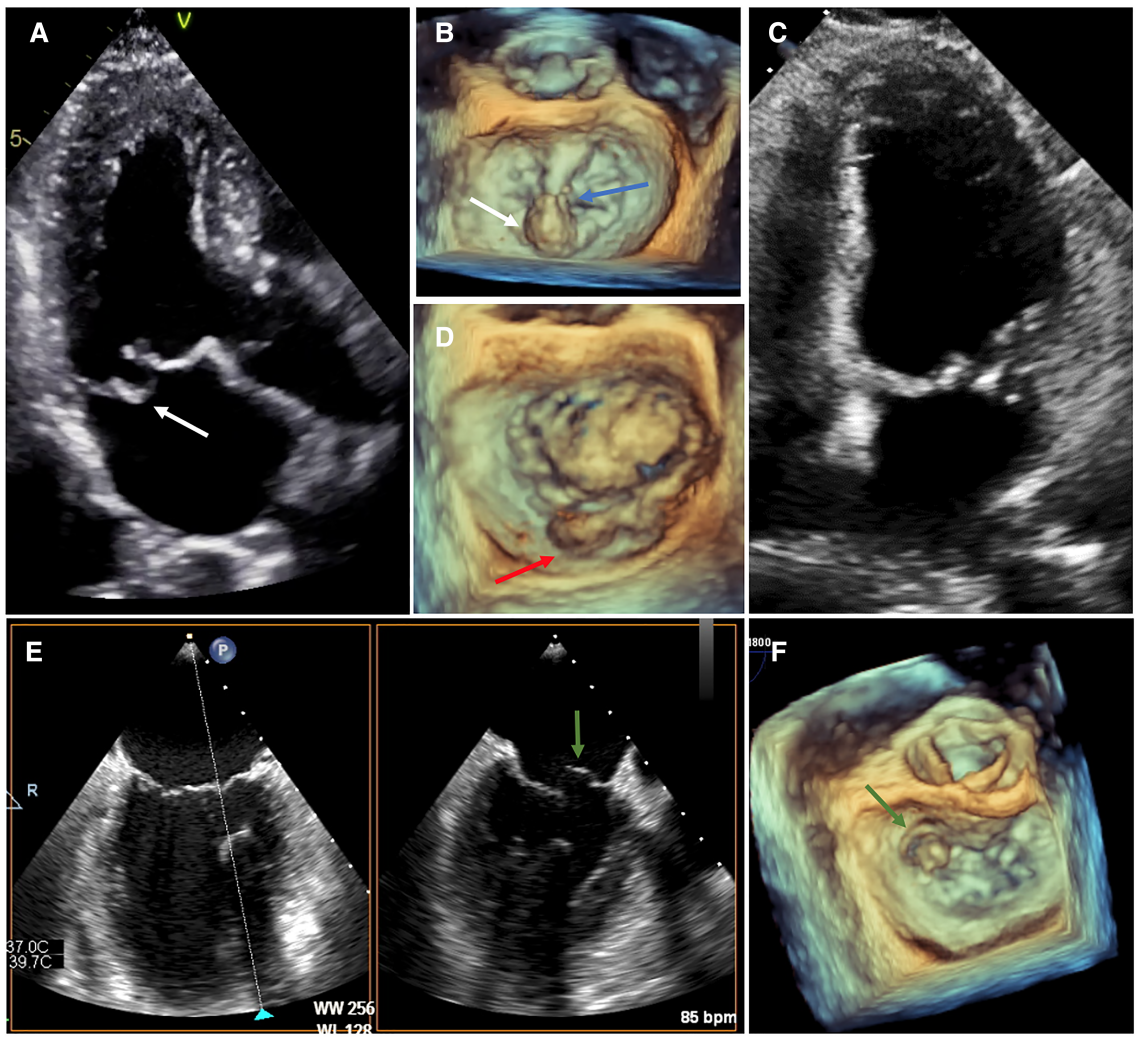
Morphological assessment of flail leaflet. (A,B) Patient with posterior flail leaflet visible on TTE (the tip is directed towards the LA). TEE confirms the involvement of segment P2 (white arrow) with chordae rupture (blue arrow). (C,D) Patient with bi-leaflet MV prolapse and P2 flail (red arrow) only visible on 3D-imaging TEE (surgical “en face” view). (E,F) Patient with flail involving the segment A1 (green arrow) visible on TEE imaging using biplane mode from the bi-commissural view (60–90°), and on 3D-imaging (surgical “en face” view). LA, left atrium; TTE, transthoracic echocardiography; TEE, transesophageal echocardiography.
